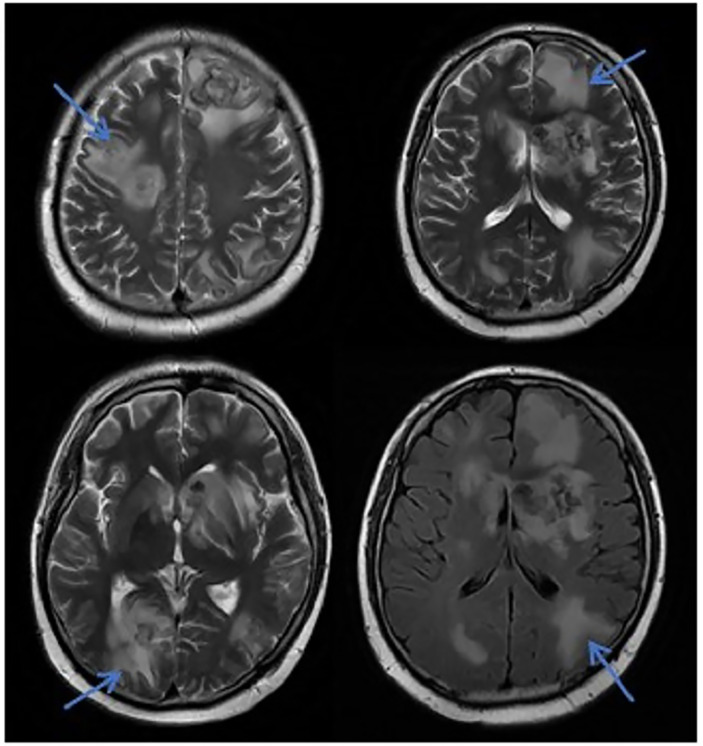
MRI in patients with CNS leukemia and infection. Primary central nervous system infiltration MRI indicated that the lesion showed mixed signals on the T1WI and nodules with mixed signals on the T2WI and T2WI flair. A DWI spot-like hyperintensity was observed in the lesion, and the enhancement.
